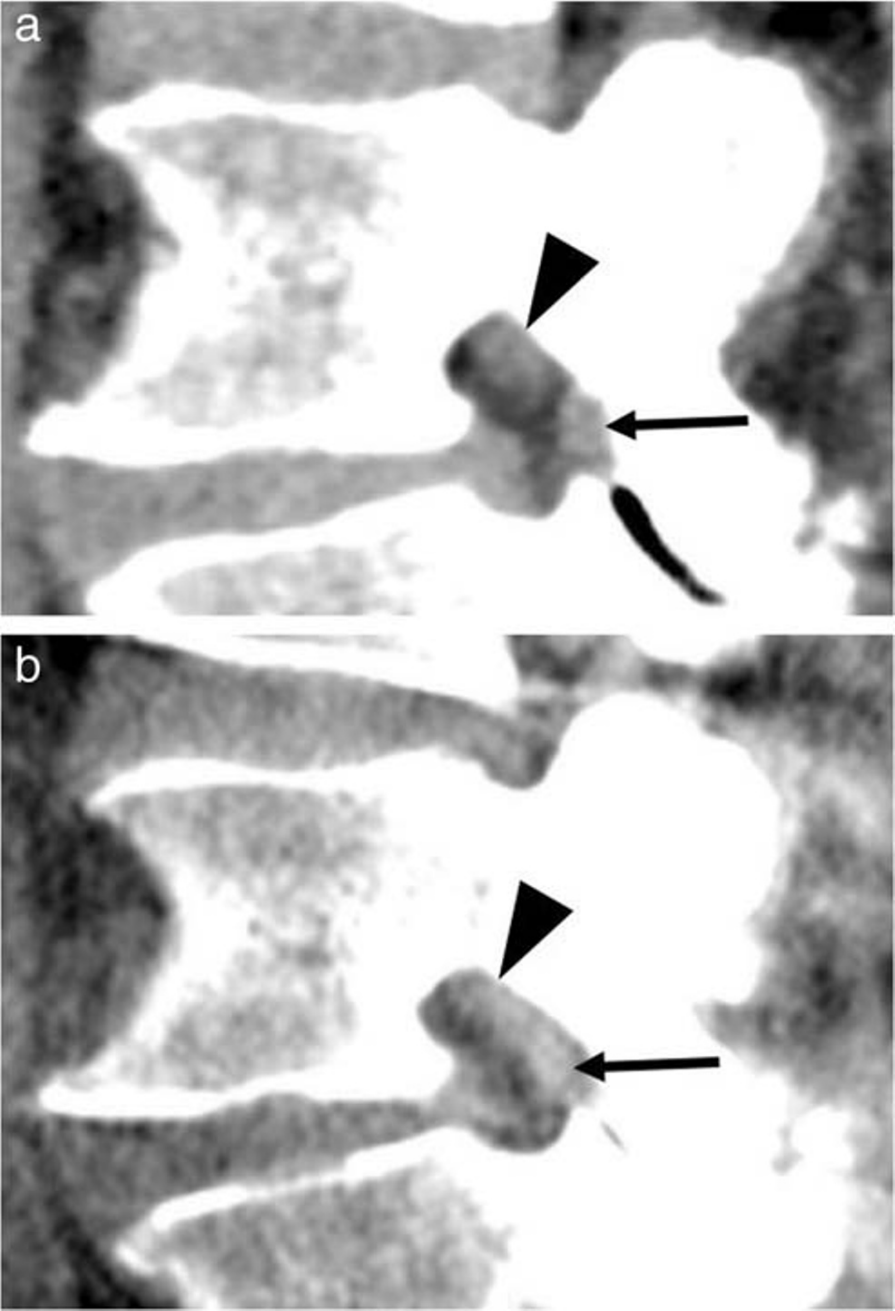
Comparison of image quality of soft tissue resolution of the neural foramen and flava ligaments on CT (a) and 3D tomography (b). Soft tissue resolution is better with CT. Note that contact of the flavum ligament (b; arrow) to the nerve root was depicted with weight-bearing. Therefore, perineural fat is obliterated to half of the nerve root circumference in standing (b; arrowhead) compared with three quarters of the circumference in the supine position (a; arrowhead). However, the nerve boundary is blurred on 3D tomography (b) compared with that on CT (a) representing the higher noise and reduced overall image quality using twin robotic X-ray
